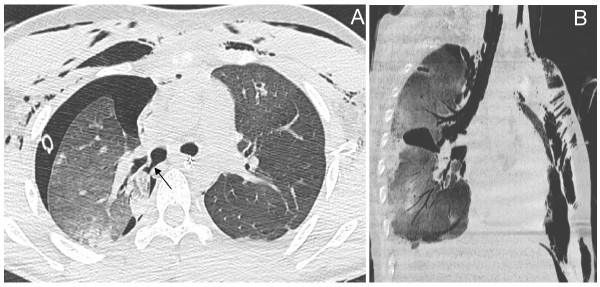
Second thoracic computed tomography scan on day 2 (axial and oblique views). (a) The intermediate trunk is disrupted with a visible posterior wall defect below the origin of the right upper lobe bronchus (arrow). Note the persisting right pneumothorax despite adequate chest tube drainage. (b) An abnormal lucency raising the possibility of a bronchial disruption is seen on the oblique view.
